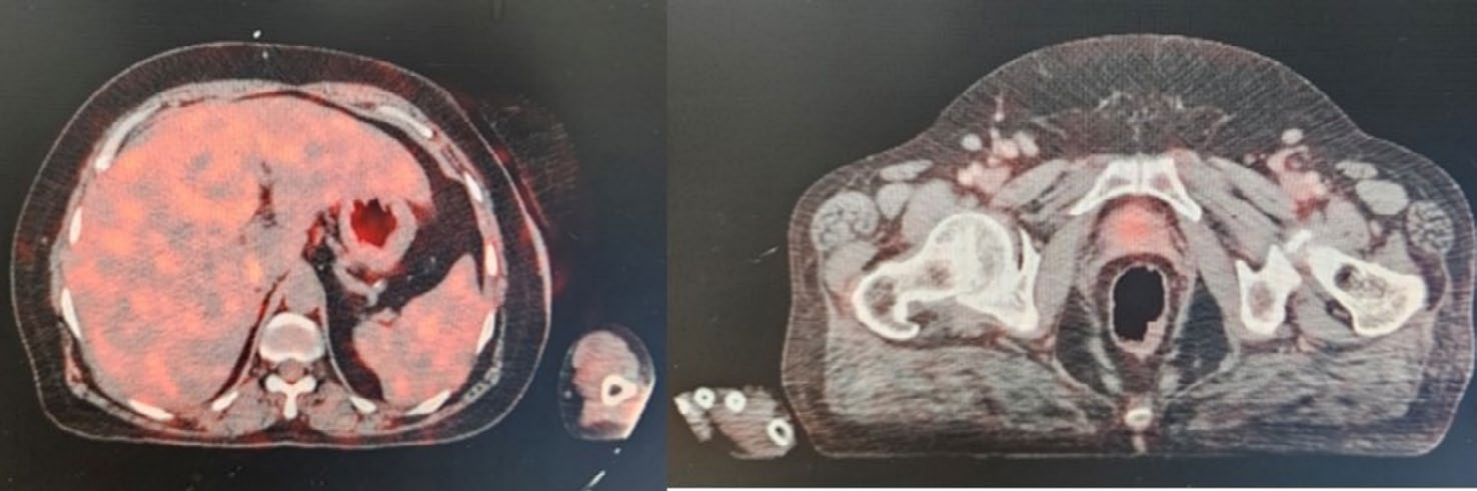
FDG 18 PET CT images demonstrating persistent complete metabolic remission (at the end of 3 years of follow‐up).
